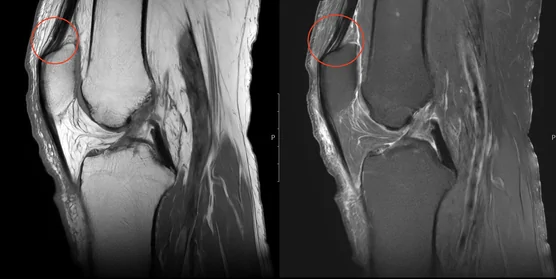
Right knee. Sagittal MRI scans of the distal quadriceps tendon complex. The left image shows a T1‐weighted sequence, whereas the right image shows a fluid‐sensitive fat‐suppressed sequence. In both images, the red circles highlight the thin fatty areolar layer interposed between the rectus femoris tendon and the deeper quadriceps tendon layers. This adipose tissue plane represents a key anatomical landmark for identification of the correct dissection plane during graft harvesting. (MRI, magnetic resonance imaging.)
